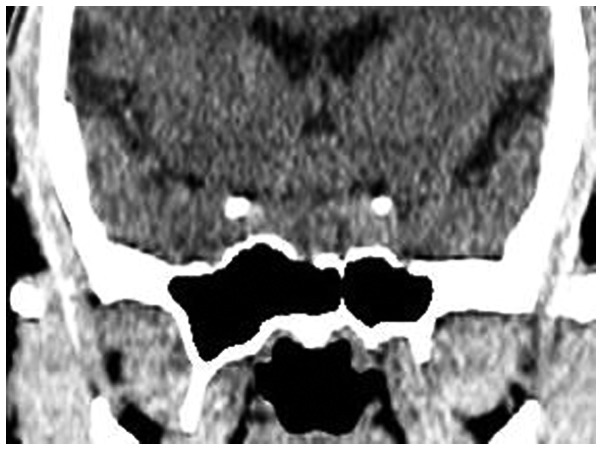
Computed tomography (CT) showing a parenchymatous mass in the sellar region, damage of the sclerotin of the anterior clinoid process, partial obliteration of the suprasellar cistern and a pressurized anterior third ventricle and precornu.
